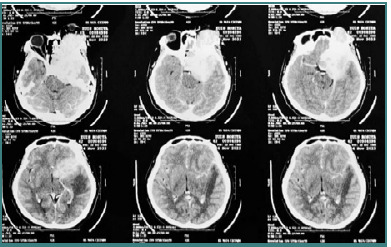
CT scan showed that the sulcus and gyri were not compressed, there was no peritumoral edema and midline shift
